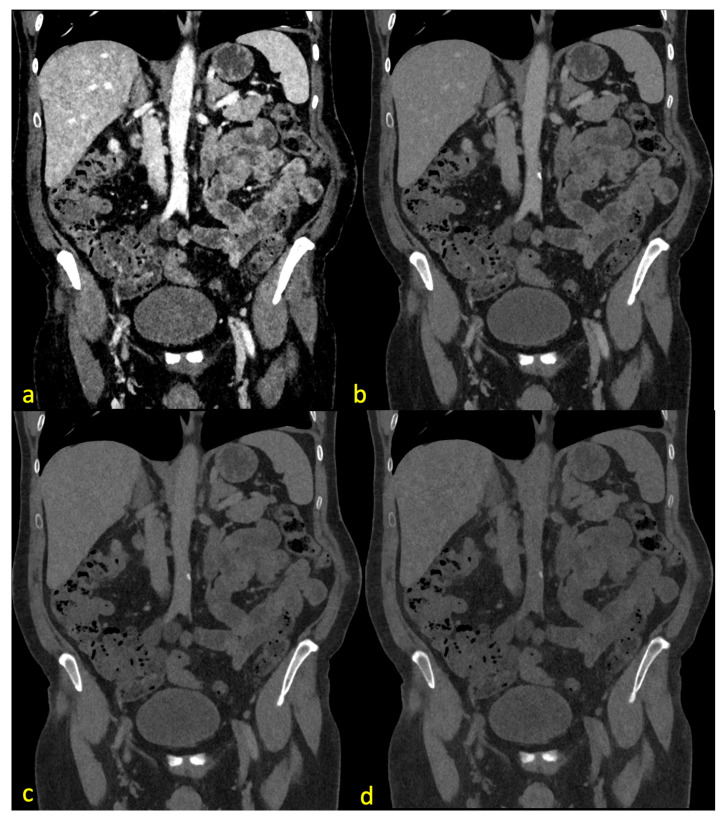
The same CT slice with pv contrast (center 130, width 550) at 40 keV (a), 70 keV (b), 100 keV (c), and 190 keV (d). (a) demonstrates major image noise with acceptable image quality and optimal vessel contrast. (b) shows no noise with a good vessel assessability and optimal image quality. (c) shows no noise, acceptable quality, and poor vascular contrast. (d) demonstrates no noise, no vessel contrast, and poor image quality.
